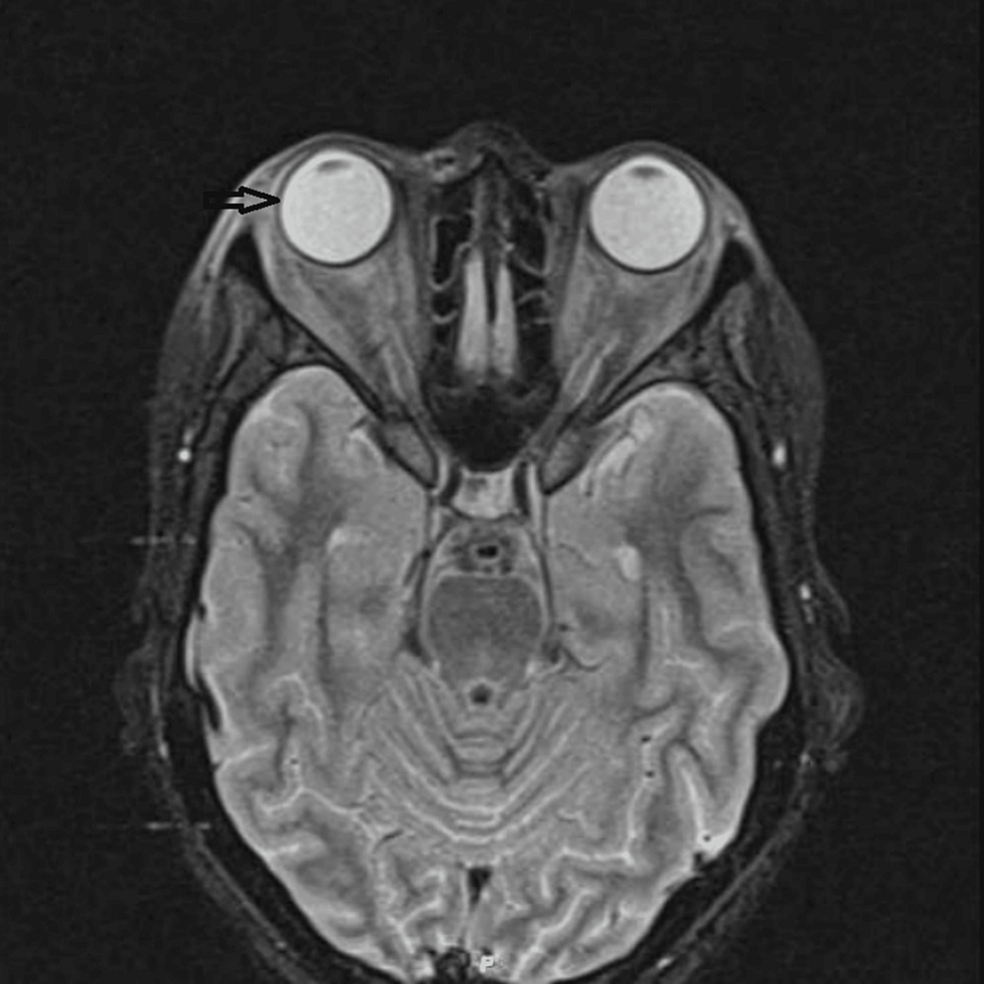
Orbital magnetic resonance imaging without contrast showing bilateral diffuse preseptal soft tissue swelling more on the right side.
